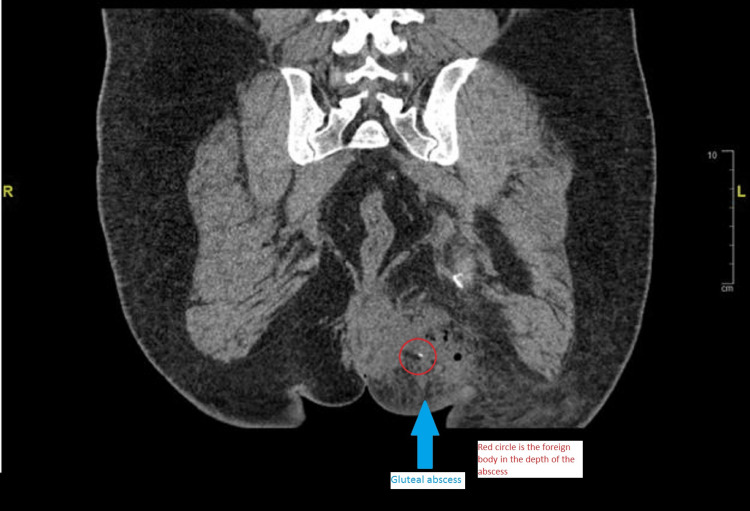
CT scan of pelvis (coronal section) showing linear foreign body (red circle) in left gluteal region abscess with gas pockets and subcutaneous fat stranding (blue arrow)
